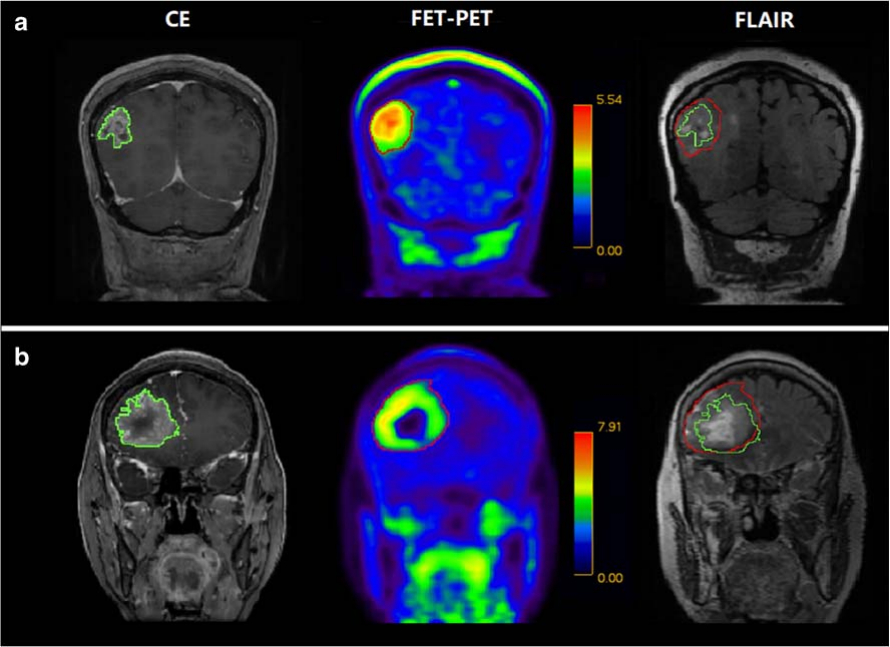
Representative CE MRI, FET-PET images, and the relationships among the abnormal areas identified on CE MRI, FET-PET, and FLAIR images. a In patient 19, *V*_PET_ was much larger than *V*_CE_. The abnormal signal areas identified on FLAIR image were larger than both *V*_CE_ and *V*_PET_ (type 1). b In patient 29, *V*_CE_ was nearly contained within *V*_PET_. *V*_PET_ was partially beyond the extent of the abnormal signal areas identified on FLAIR image (type 2)
